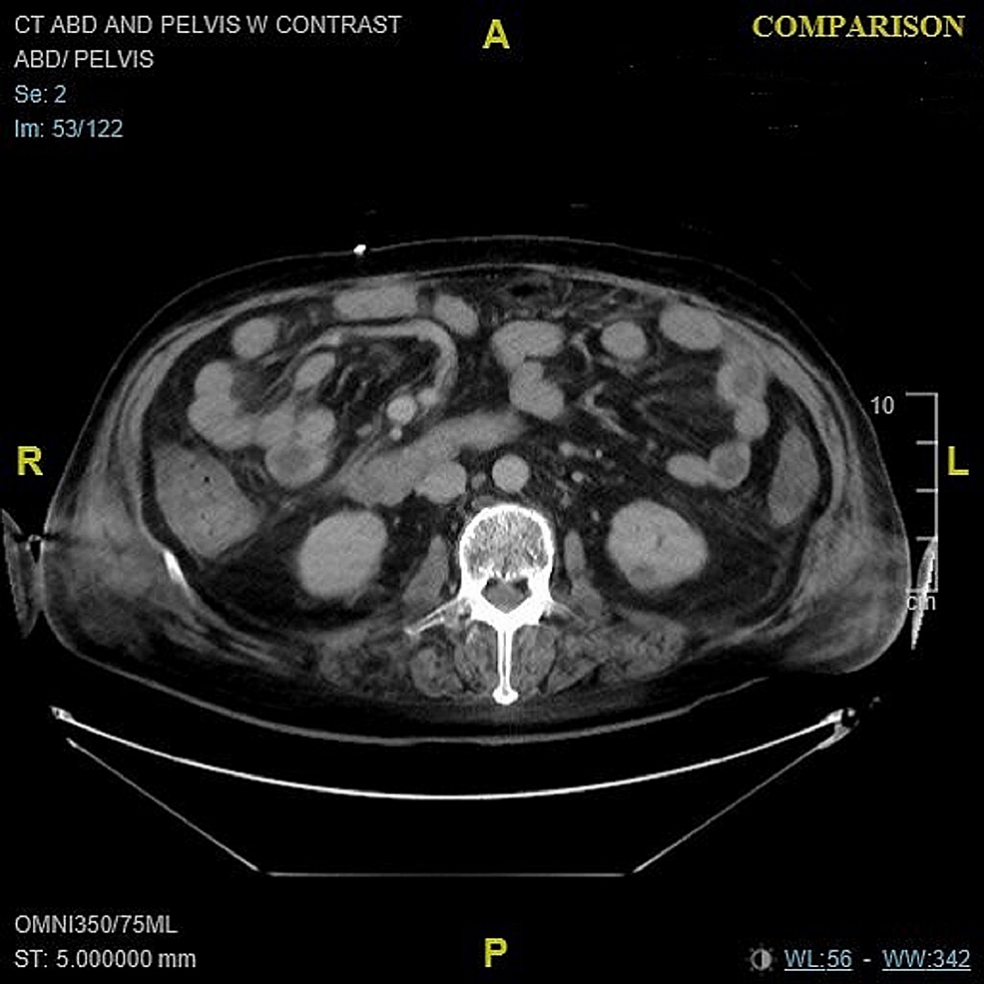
CT of the abdomen/pelvis on admission. Axial view: On admission, marked diffuse colonic wall thickening with adjacent fat stranding. CT: computed tomography
